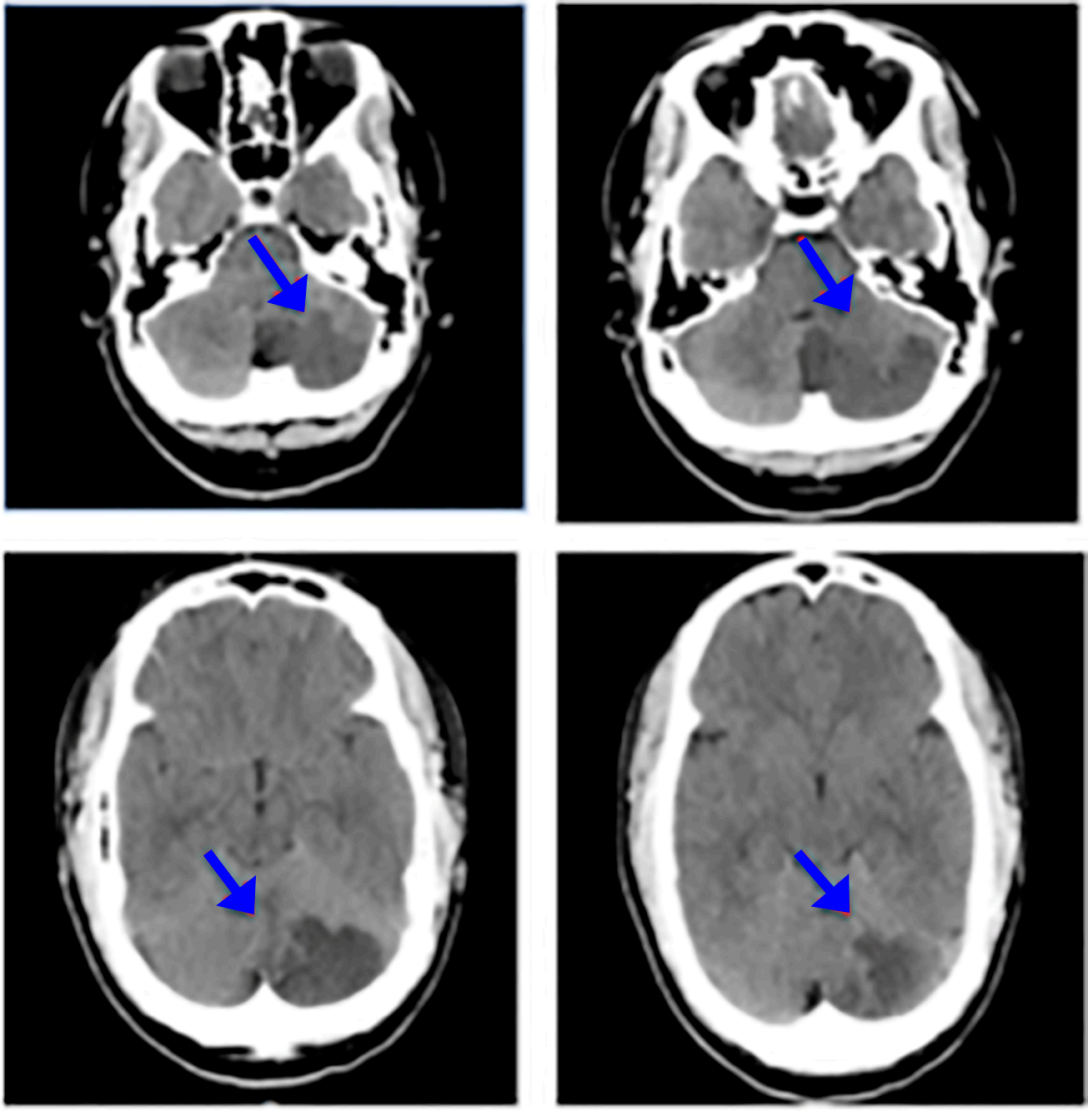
CT Demonstrating an Acute Left Cerebellar Infarct in the Distribution of the Posterior Inferior Cerebellar Artery. Pictures are from Caudal (top left) to Cranial (Bottom Right) Direction.
